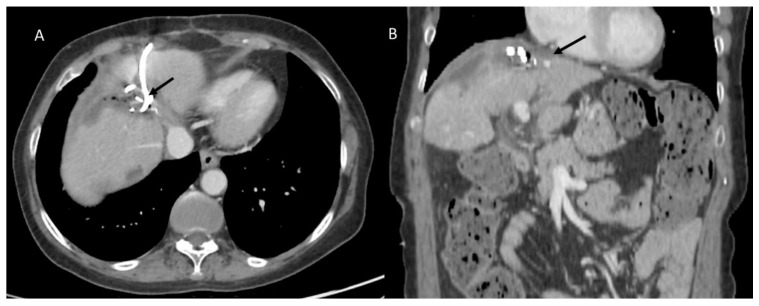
CT-guided hepatic infected biloma drainage (arrows) and postprocedure assessment in portal phase of contrast study in axial (A) and coronal (B) plane.
